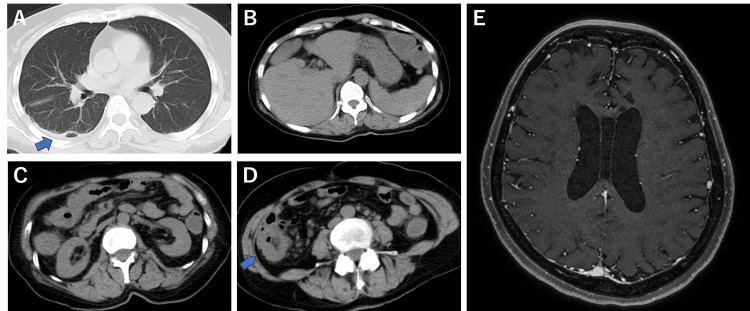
Imaging tests during the second hospitalization (days 126-171) (A) Pulmonary computed tomography (CT). (B-D) Abdominal CT. (E) Contrast-enhanced magnetic resonance imaging (MRI).
